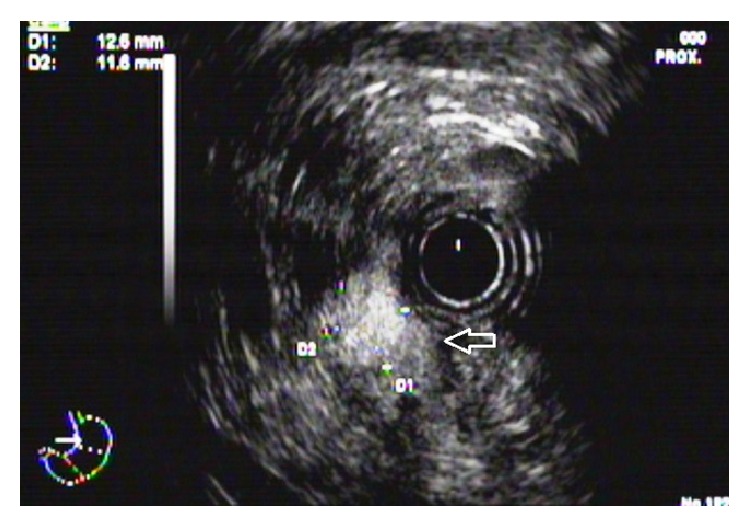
Endoscopic ultrasound image of a 12.5 × 11.6 mm hypoechoic mass located at pancreatic head.
